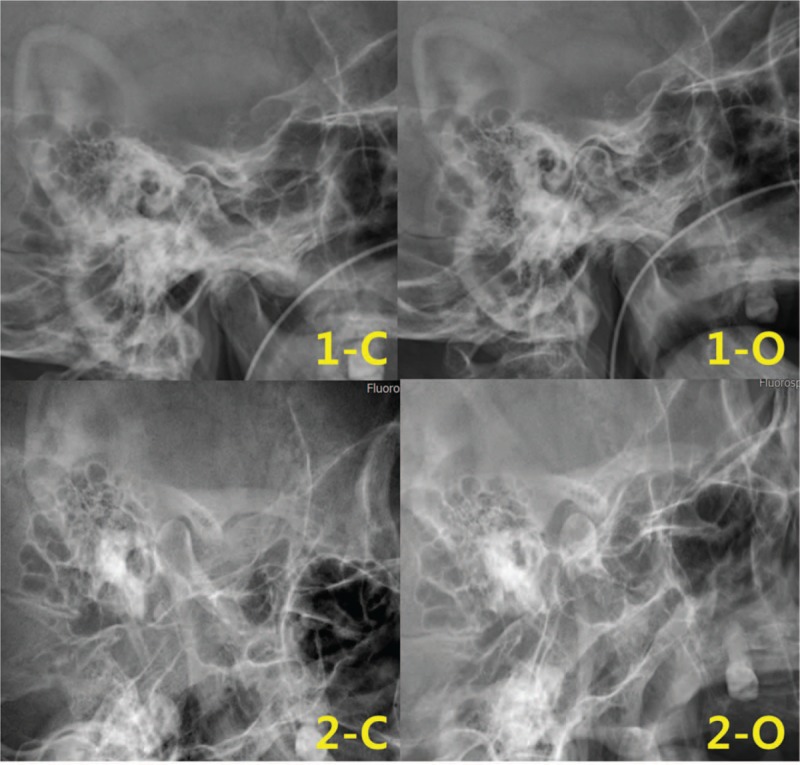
Left temporomandibular joint X-ray. (1) The left mandibular condyle remained in the mandibular fossa even when she attempted to open her mouth widely (May 17, 2017). (2) The left mandibular condyle was slightly translated to the mandibular fossa and moved forward to the articular tubercle (June 7, 2017). C = close, O = open.
